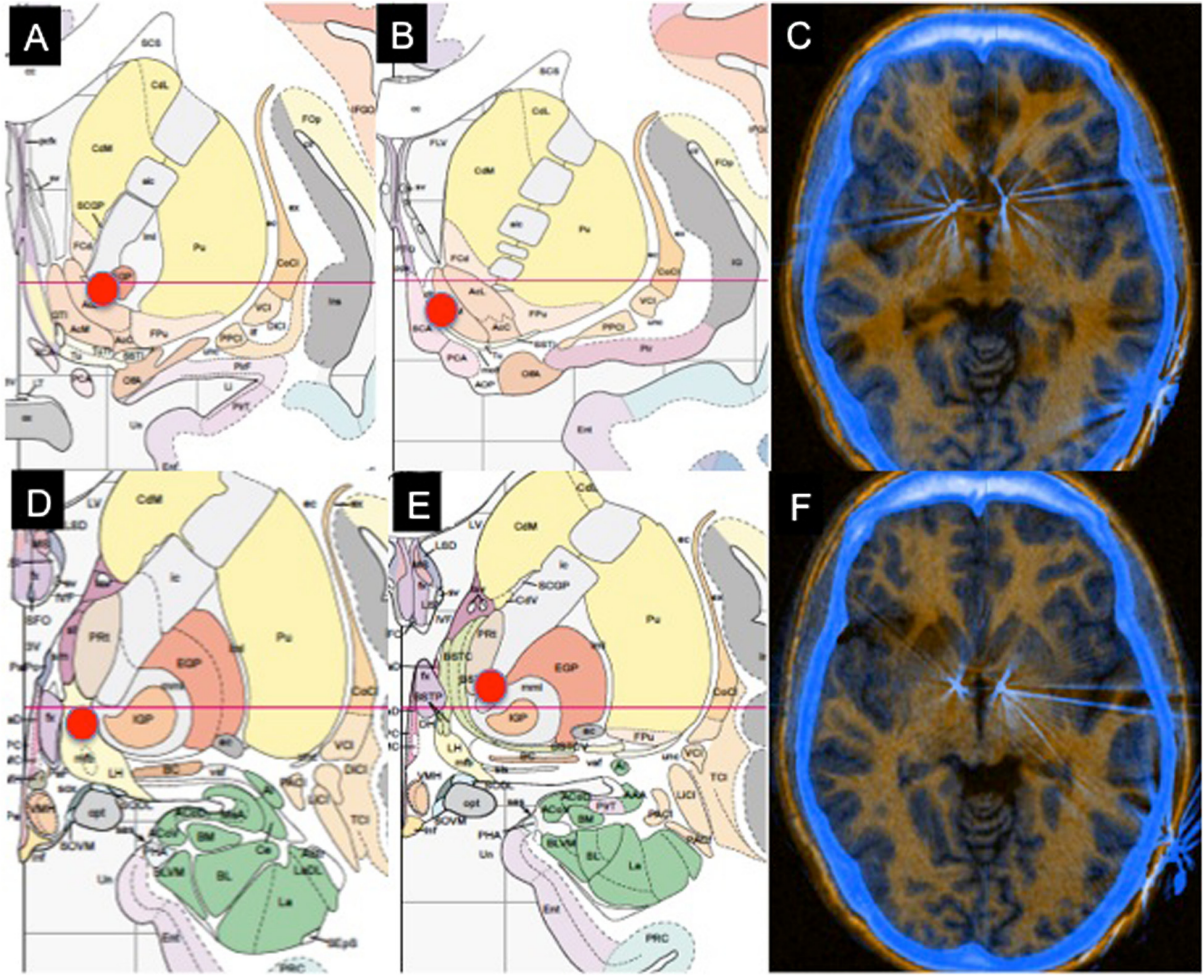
Anatomical location of the stimulation points and postoperative location of the electrodes on both surgeries. Red dots (A,B,D,E) show the position of the center of the stimulation after the first and second surgery superimposed in Mai atlas*†. a Coronal section 4,2 mm anterior to AC showing the middle point between the two active contacts on the right side after the first surgery. b Coronal section 5,8 mm anterior to AC showing the middle point between the two active contacts on the left side after the first surgery. c Postoperative CT fused with MRI showing the radiological position of the electrodes with respect to anterior comissure after the first surgery. d Coronal section 4,0 mm posterior to AC showing the middle point between the two active contacts on the right side after the second surgery. e Coronal section 2,7 mm posterior to AC showing the middle point between the two active contacts on the left side after the second surgery. f Postoperative CT fused with MRI showing radiological position of the electrodes with respect to anterior comissure after the second surgery.  Images on both sides correspond to frontal sections of left hemispheres of the human brain in Mai atlas. Frontal sections most closely related with anatomical location of the stimulation point have been chosen for the figure. † Elsevier Ltd. granted written permission to use, adapt and publish the images belonging to Atlas of the Human Brain, 3° ed (ISBN 9780123736031), Mai et al.*
